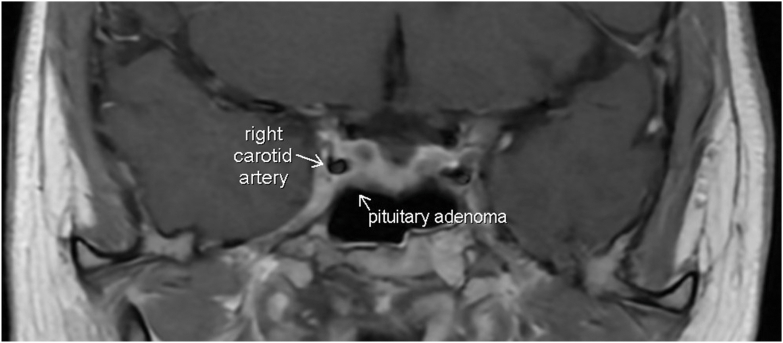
MRI pituitary coronal image revealing *right* eccentric heterogenous enhancing sellar mass which is decreased in size. Redemonstrated residual tissue around the *right* carotid artery.
